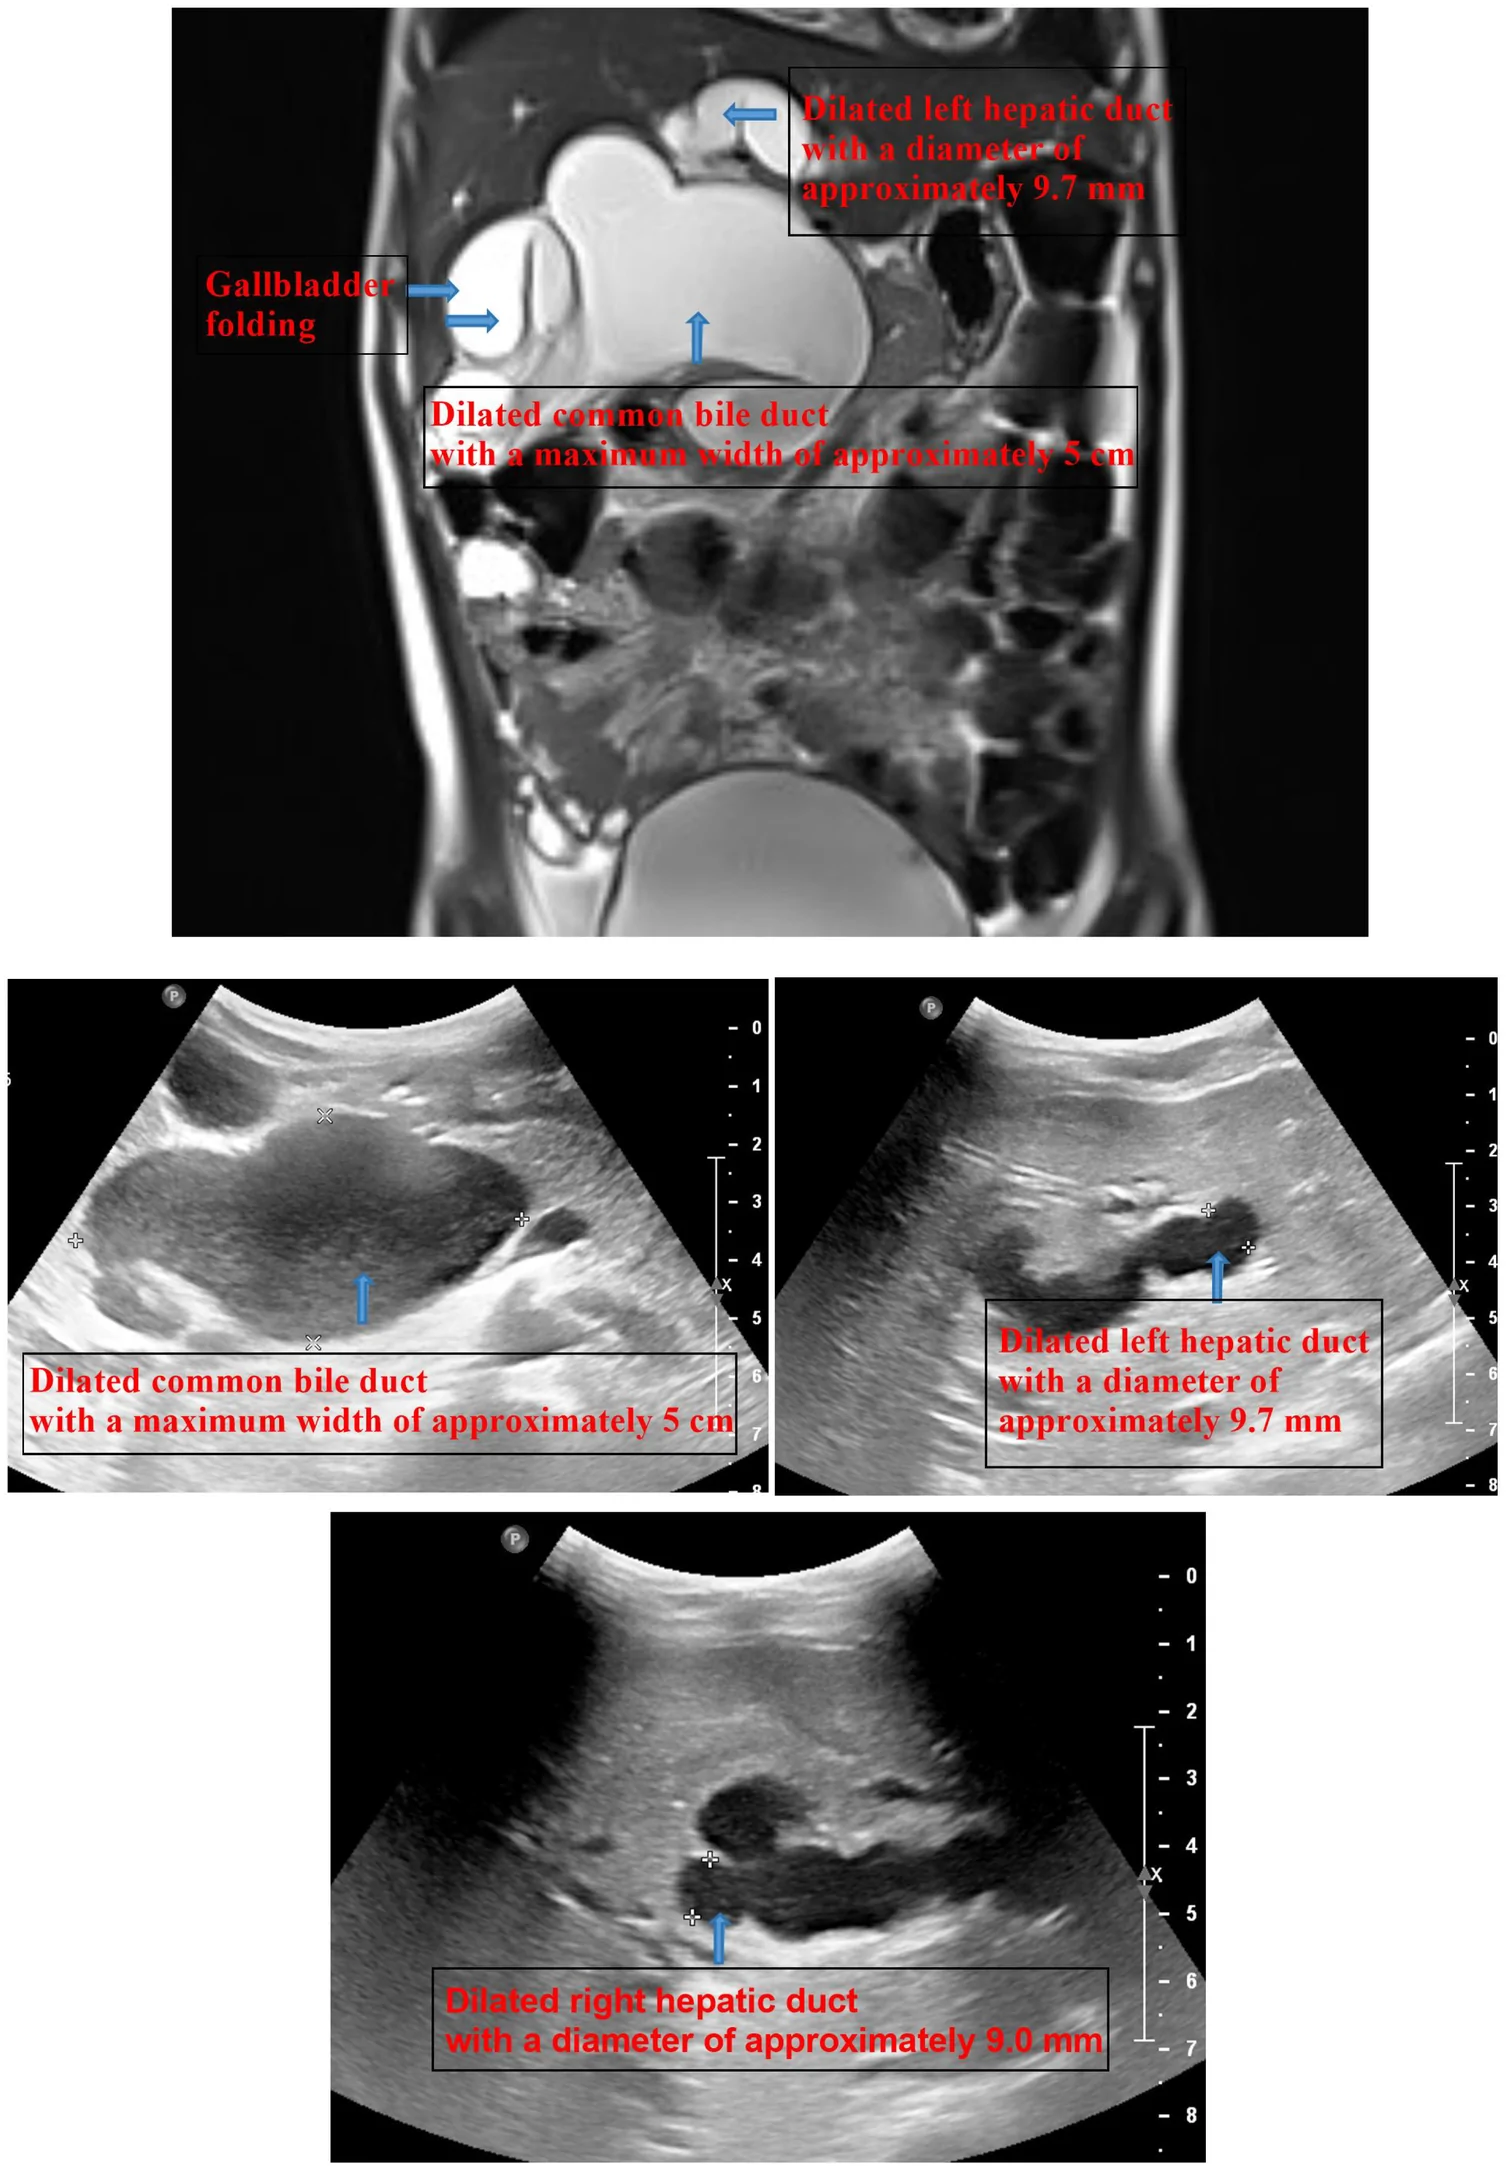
Severe extensive dilatation of intrahepatic bile ducts plus cystic biliary stasis/surrounding inflammatory changes (severe case of type IVa). Imaging findings of severe intrahepatic bile duct dilatation and biliary sludge deposition in advanced Todani type IVa choledochal cyst. Top row: Coronal MRCP images show massive cystic dilatation of the common bile duct (maximum diameter 5 cm), marked dilatation of bilateral intrahepatic bile ducts with a beaded appearance, gallbladder folding and tortuous cystic duct, and mild pancreatic duct dilatation. Bottom panels: Corresponding US scans confirm giant extrahepatic cystic dilatation and dilated left/right hepatic ducts; sonographic report demonstrates biliary sludge within the cyst and gallbladder inflammatory changes. Pericystic inflammatory infiltration and mild perihepatic free fluid are noted on MRI. All dilated biliary segments are annotated with arrows and dimension labels.
